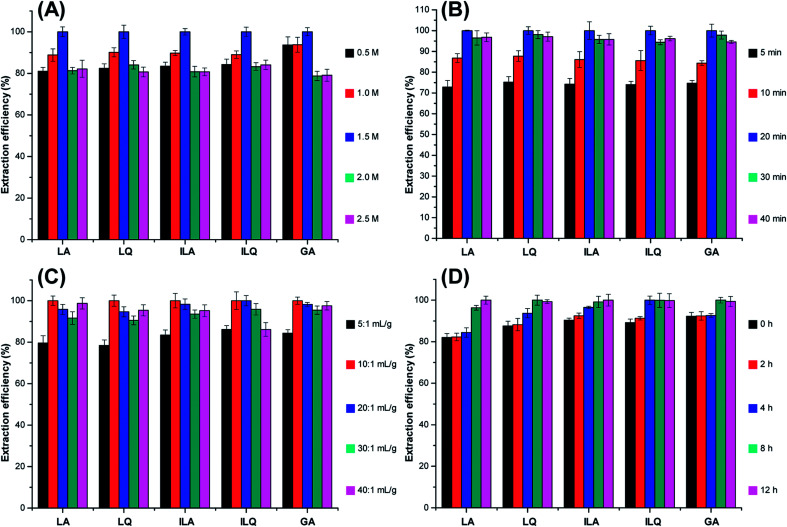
Effect of [C_4_MIM]Ac concentration (A), ultrasonic time (B), solvent to solid ratio (C) and soaking time (D) on the extraction efficiency of five target analytes from licorice. The extraction efficiency is expressed as the percent of observed values to max observed value of each target analyte.
